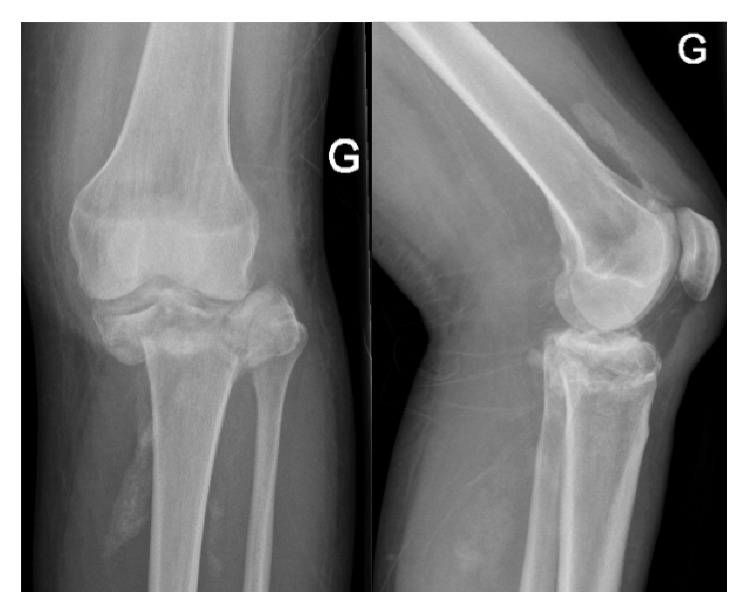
Initial X-rays of the left knee.
